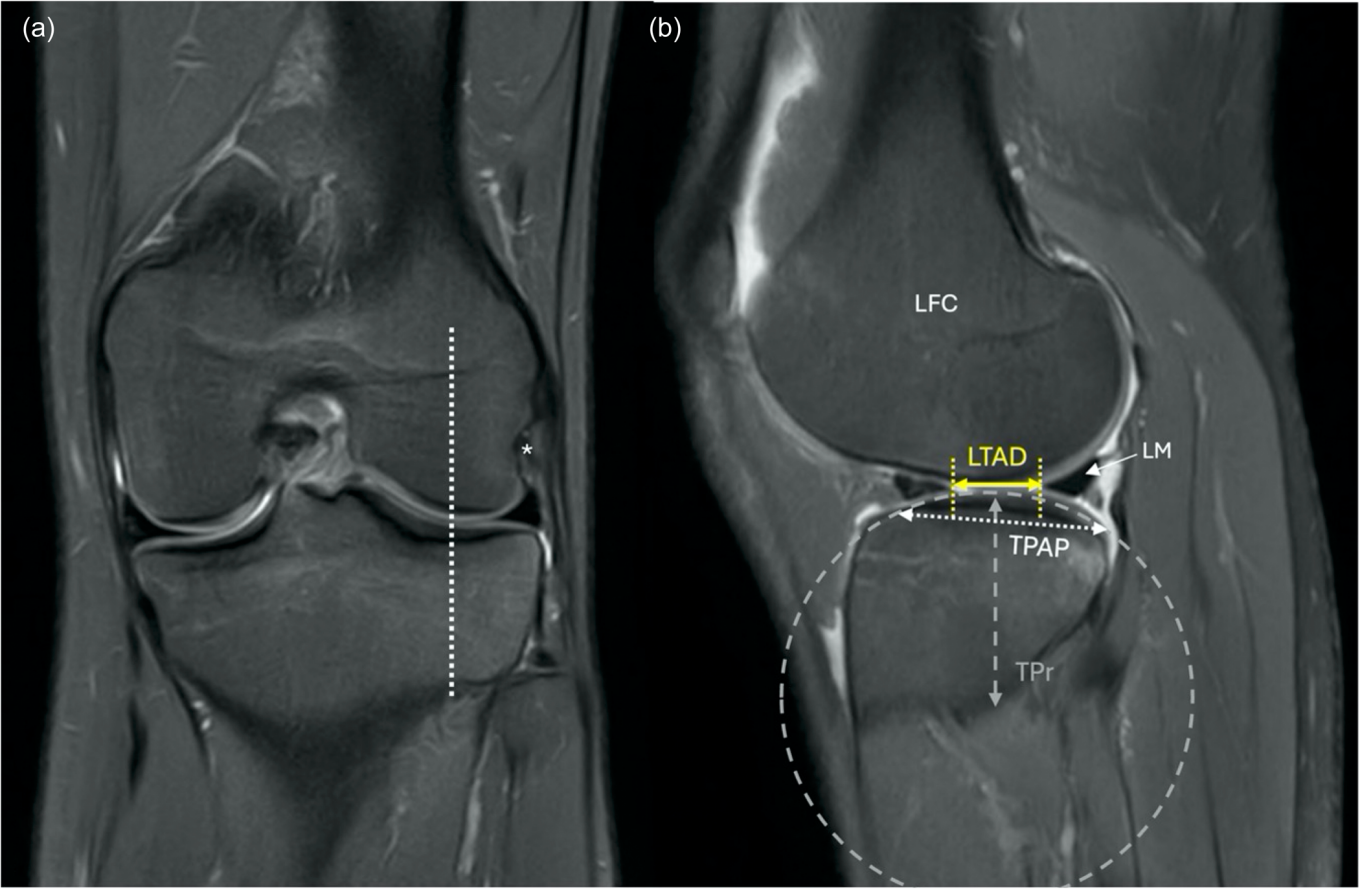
(a) The midsagittal plane of the lateral femoral condyle (LFC) is located at the level of the popliteal femoral insertion point (*) on a coronal magnetic resonance image. Identification of the midsagittal plane of the femur involves locating the vertical axis (represented by the dashed white line) that intersects the most inferior point of the convex surface of the articular cartilage at the distal femur. This axis generally aligns close to the midpoint of the mediolateral articular width on the lateral side of the joint. (b) The anteroposterior distance between the most posterior point of the anterior horn and the most anterior point of the posterior horn of the lateral meniscus (LM) is referred to as the lateral tibiofemoral articular distance (LTAD). The tibial plateau anteroposterior length (TPAP) represents the length of the articular surface measured along the subchondral plate. To characterize the curvature of the proximal tibial articular surface, a best‐fit circle (grey) is superimposed. The tibial plateau radius of curvature (TPr) is defined as the radius of this circle. A smaller LTAD, a shorter TPAP and a smaller and more convex TPr are potential risk factors for ACL injury. ACL, anterior cruciate ligament.
